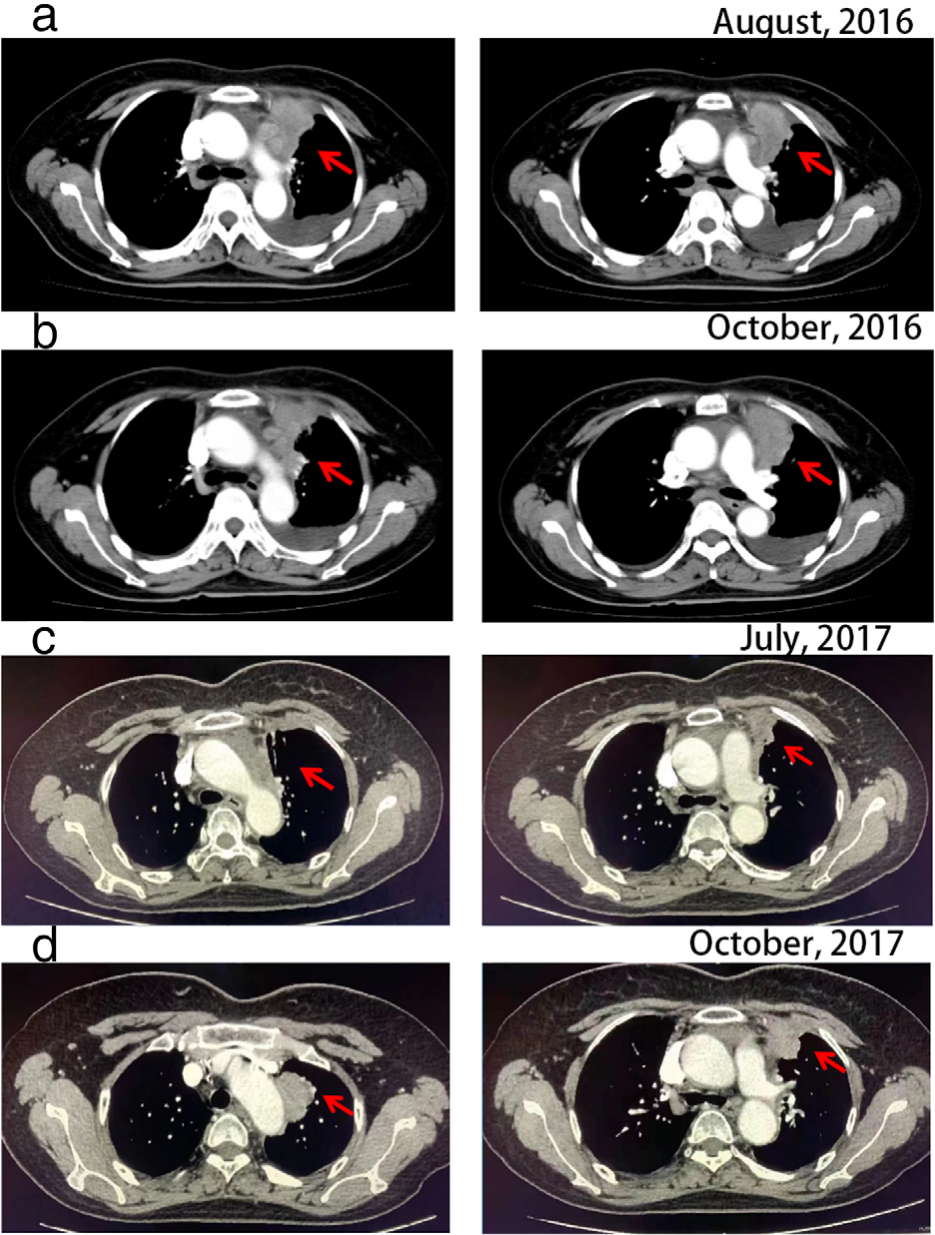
Computed tomography (CT) images of the patient's primary pulmonary tumor lesion (a) before chemotherapy (August 2016), (b) resistant to chemotherapy (October 2016), (c) response to brigatinib (July 2017), and (d) resistant to brigatinib (October 2017), respectively. The red arrows indicate the pulmonary lesion
